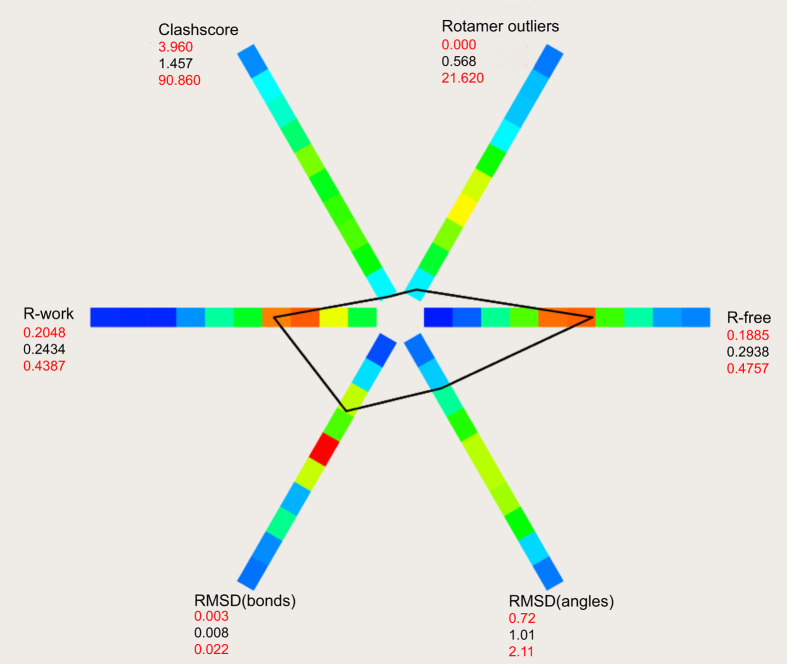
POLYGON analysis of the quality statistics of the rhodopsin-arrestin complex structure. A comparison of the rhodopsin-arrestin complex structure with 535 structures in the PDB at comparable resolution indicates that the quality of the current structure is equal to or better than most X-ray structures in the database of similar resolution.
